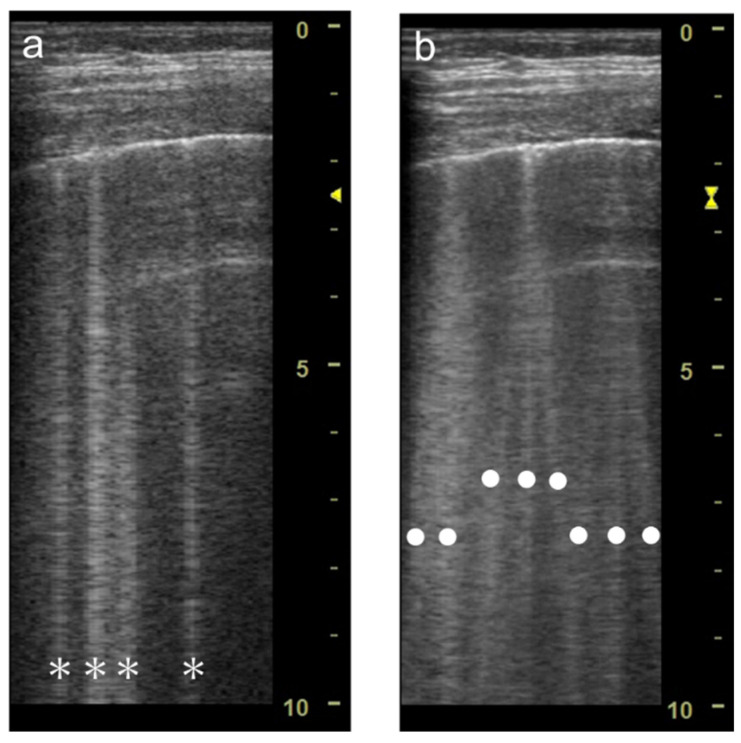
Ultrasound images obtained by LOGIQ V scanner (GE Healthcare) with a linear transducer without (a) and with spatial compound imaging (b) in cardiogenic pulmonary edema. With spatial compound imaging enabled, each single B-line (asterisks) changes to multiple lines (dots) radiating from the same point on the pleural line.
